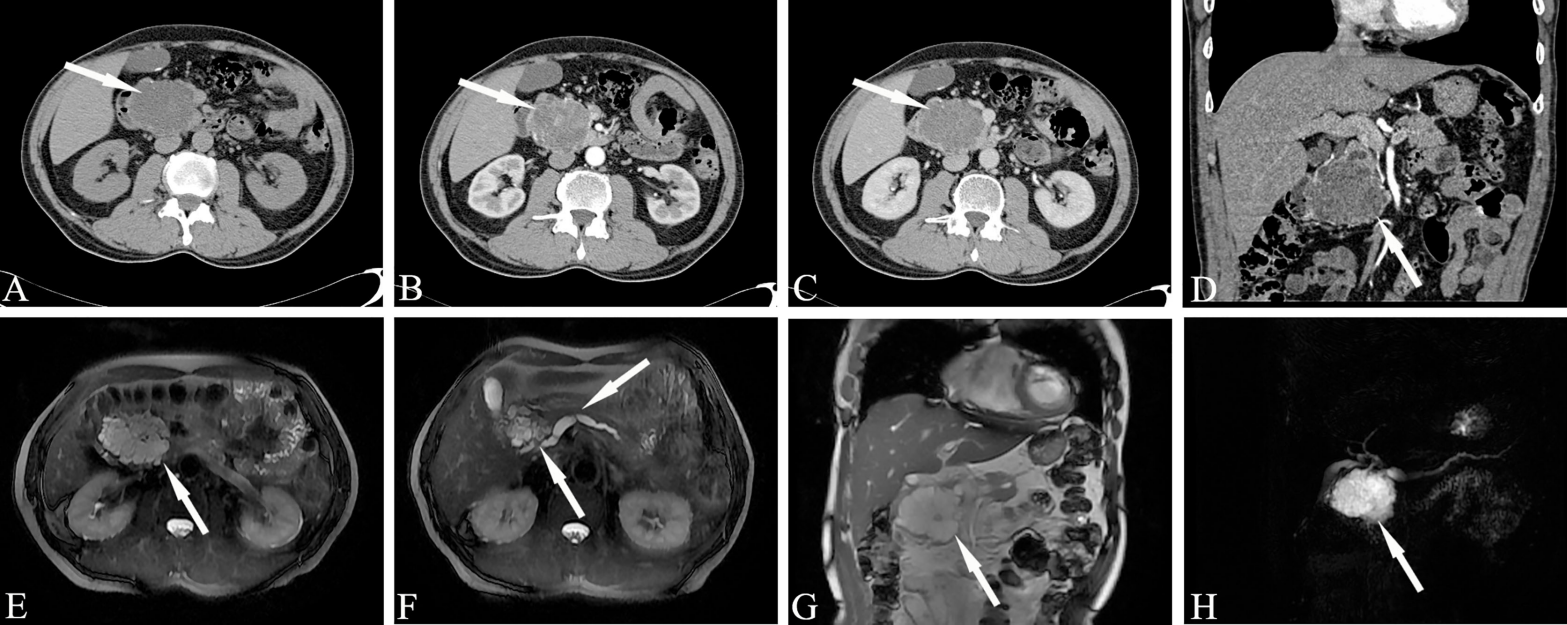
Serous microcystic adenoma, SMA (Male, 65y, physical examination revealed a pancreatic mass for one month). The sequence distribution of images is the same as that in Figure 2. (A–D) Polycystic or honeycomb cystic foci in the head of the pancreas, with a lobulated outline, like a collapsed wall dumping to the center, and slightly continuous enhancement of the cyst wall and septum. Punctate calcification can be seen in the capsule wall. The enhanced scan shows progressive medium-strength enhancement of the central scars. (E–H) Magnetic resonance imaging reveals microcapsule-like structures more clearly and the MPD was slightly dilated. A stellate scar can be seen in the center of the lesion. The white arrows in the Figures only indicate the location of the neoplasm.
